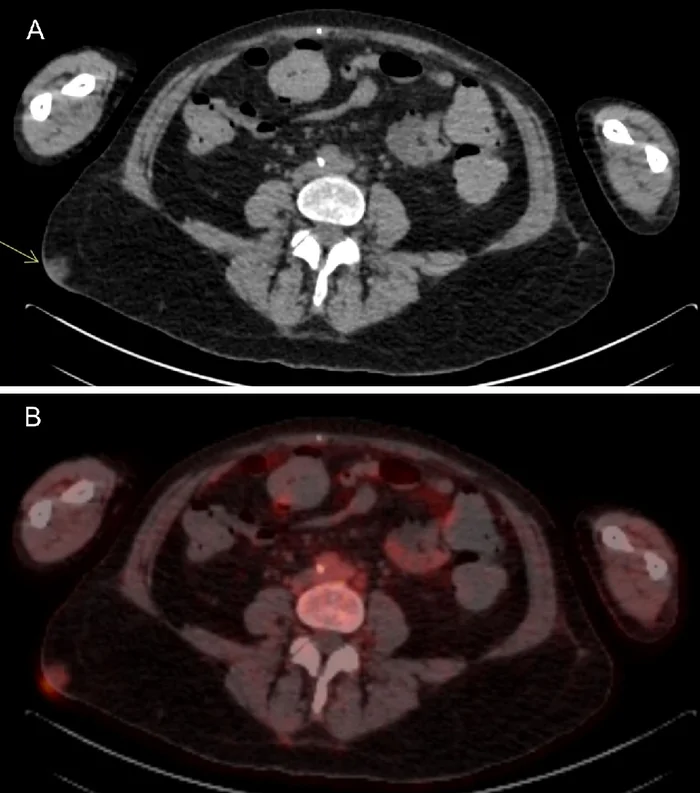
(A) Axial noncontrast-enhanced computed tomography scan shows a cutaneous-subcutaneous lesion in the right lateral lumbo-gluteal region. (B) Corresponding positron emission tomography image shows increased metabolic activity of the same lesion.
